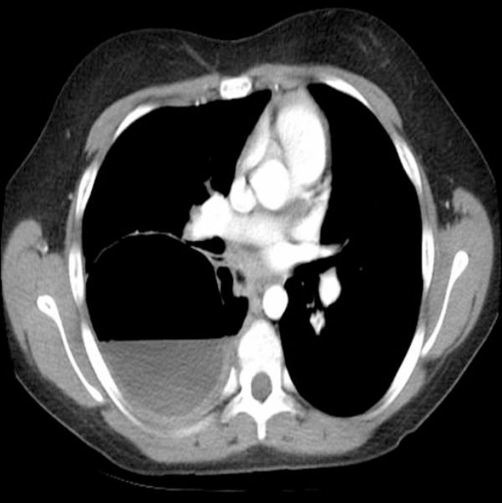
Chest CT shows bronchogenic cyst extending the entire width of the right hemithorax and approximately 50% full of fluid.
